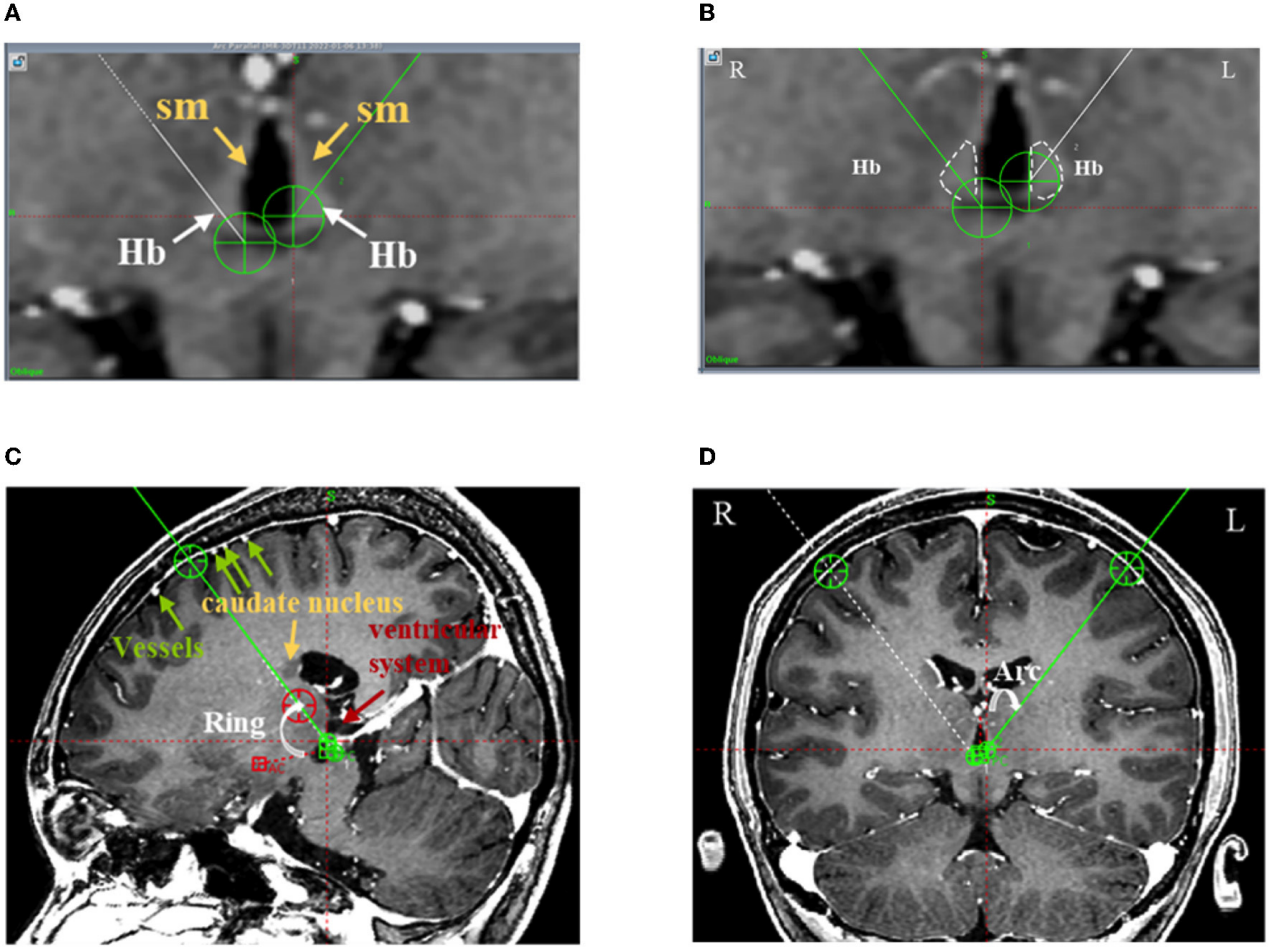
The frontal trajectory to the left LHb was planned using 3D T1 angiography images in Case 4. (A) The Hb and its afferent fiber tract (stria medullaris, sm) clearly distinguished from the surrounding gray matter and cerebrospinal fluid in the T1-weighted image; (B) The bilateral Hb delineated in white and the DBS electrode targeting the center of the left Hb; (C) The entry point of the planned frontal trajectory on the coronal plane, avoiding the cortical vessels, caudate nucleus, and ventricular system. Additionally, the planned frontal trajectory to the left side was at an angle of 59.10° relative to the AC-PC level on the sagittal plane (Ring); (D) The planned frontal trajectory to the left side was at an angle of 37.70° relative to the midline of the sagittal plane (Arc).
